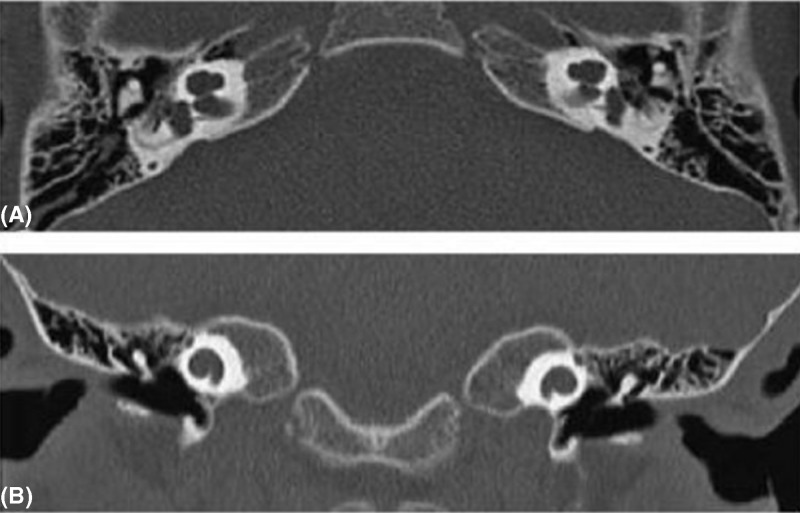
High-resolution temporal bone CT examination of the 63 patients with SLC26A4 mutation revealed MD in 15 patients CT image examples: (A) axial and (B) coronal.
